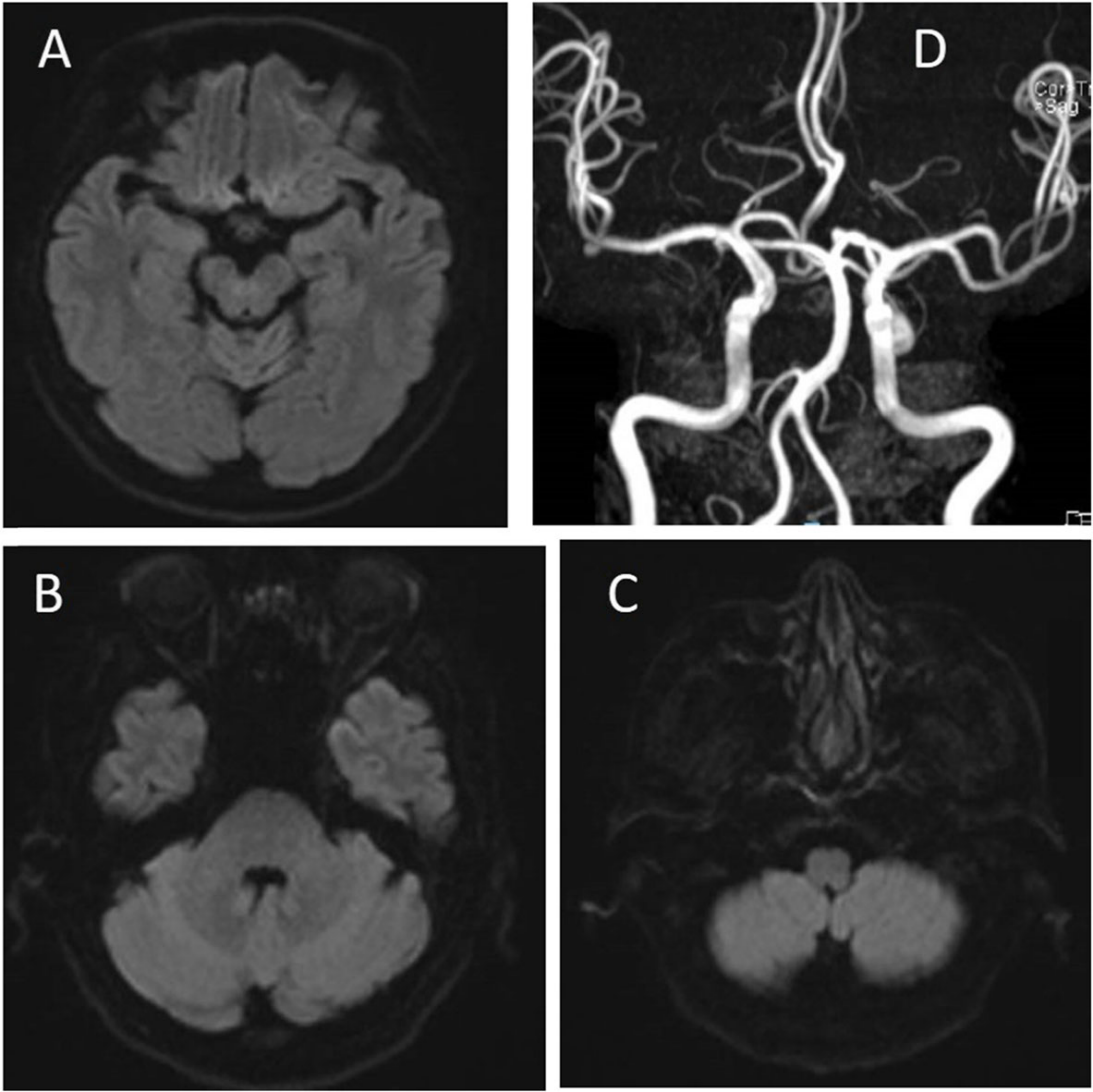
Magnetic resonance imaging of the brain showing lack of diffused weighted hyperintensities of (a) midbrain (b) pons (c) medulla. (d) normal magnetic resonance angiography
